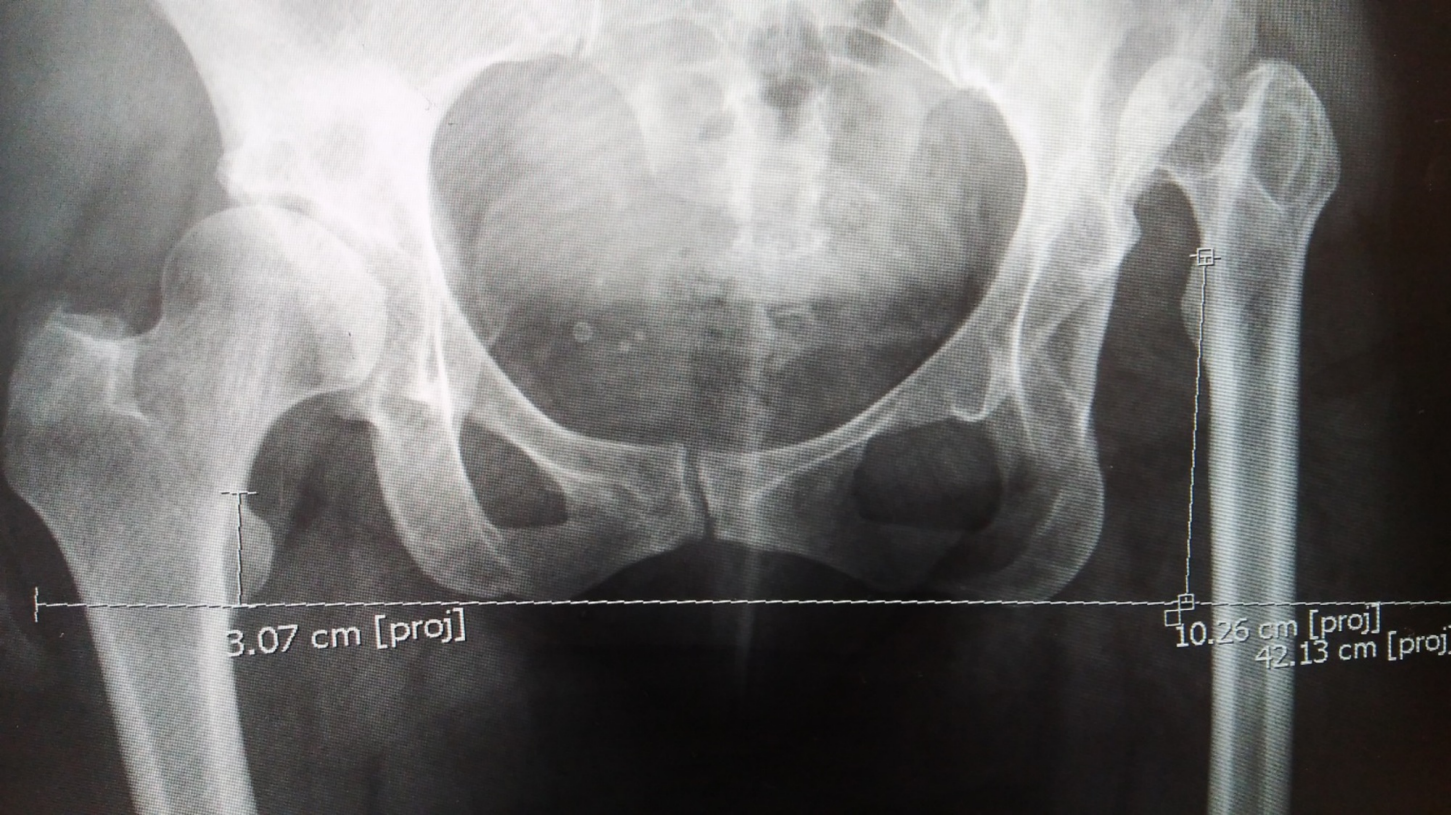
X-ray of the pelvis Pre-operative X-ray showing hip dysplasia and leg shortening.
